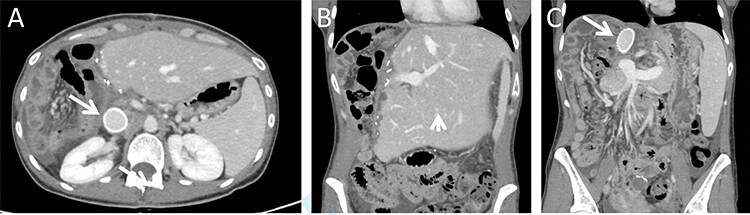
Postoperative CT imaging. (A) Axial CT image depicting patent IVC graft (arrow). (B + C) Coronal images depicting hypertrophied remnant liver (arrowhead), patent portal vein and IVC graft (arrow).
